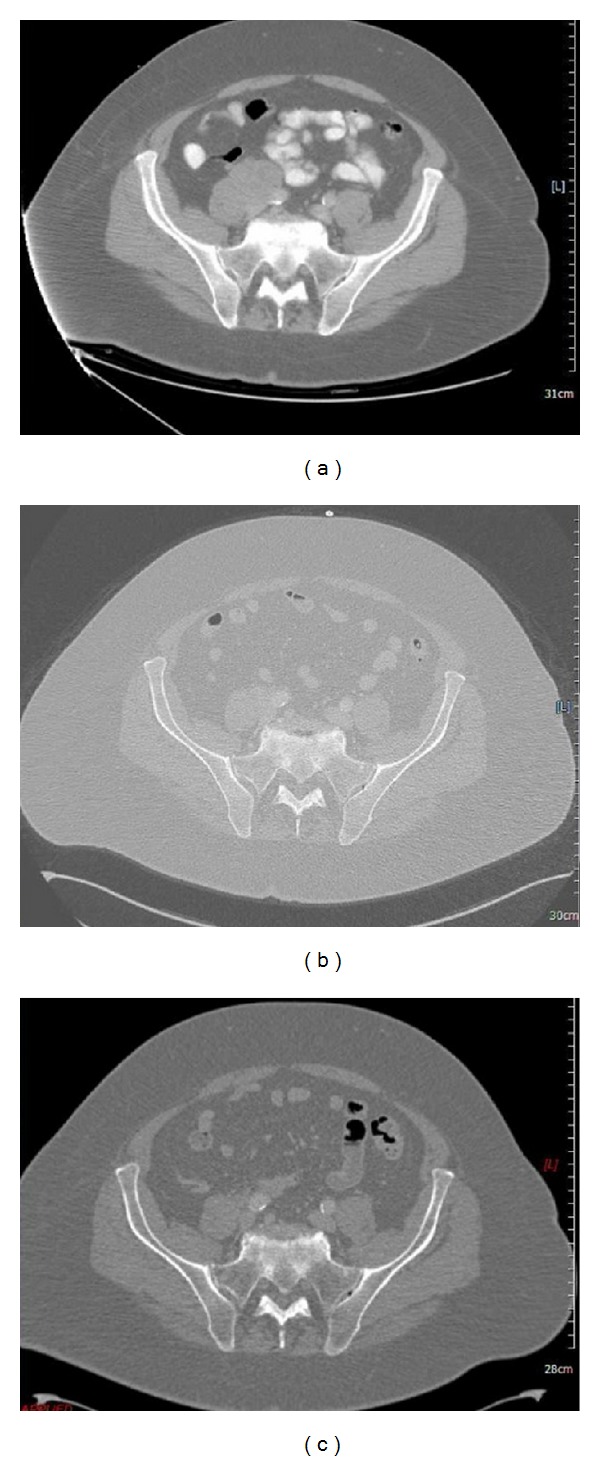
CT scan of pelvis showing right mass at diagnosis, after-chemotherapy and after-radiotherapy.
